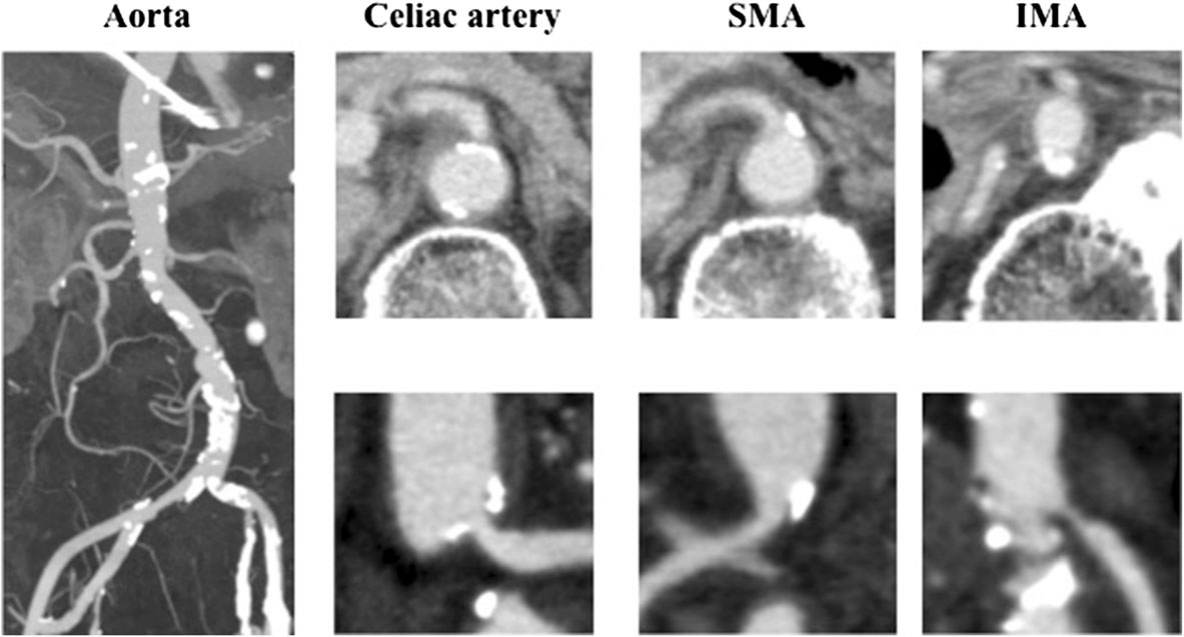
Enhanced computed tomography showed severe atherosclerosis of the arteries. The aorta showed broad calcifications, and the celiac artery showed moderate stenosis, although the lumen of the SMA was relatively patent. In addition, stenosis of the IMA was very severe. *IMA* inferior mesenteric artery, *SMA* superior mesenteric artery
